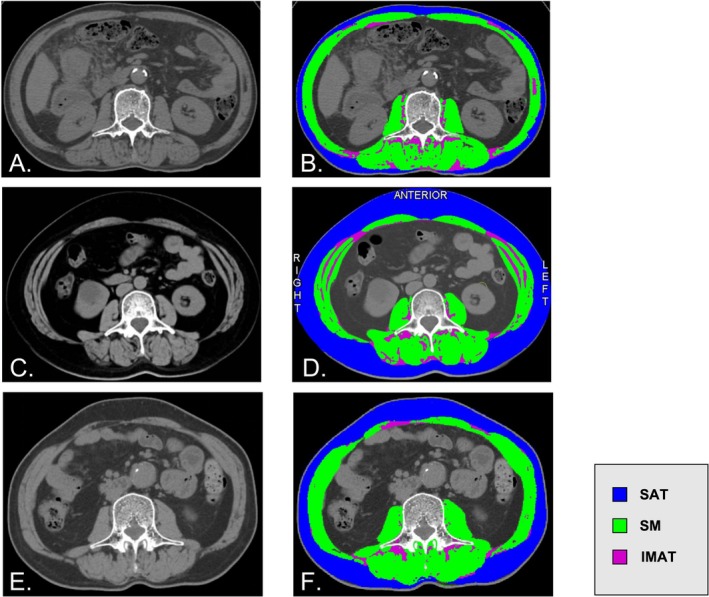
CT‐based IMAT imaging across metabolic and oncologic disease contexts. (A) Original axial CT image of a 52‐year‐old female with diabetes mellitus, obtained at a standard soft‐tissue window for body composition evaluation. (B) Corresponding segmented image with colour‐coded SAT, SM and IMAT. (C) Original axial CT image of a 72‐year‐old male with colorectal cancer, acquired at a soft‐tissue window for baseline body composition review. (D) Corresponding segmented image with colour‐coded SAT, SM, and IMAT. (E) Original axial CT image at the L3 level of a 63‐year‐old male with lung cancer, without apparent artefact or anatomic distortion. (F) Corresponding segmented image with colour‐coded SAT, SM and IMAT. Cross‐sectional areas were segmented and quantified using SliceOmatic software (Version 5.0; Tomovision, Montreal, Quebec, Canada). CT = computed tomography; IMAT = intermuscular adipose tissue; L3 = third lumbar vertebral level; SAT = subcutaneous adipose tissue; SM = skeletal muscle.
